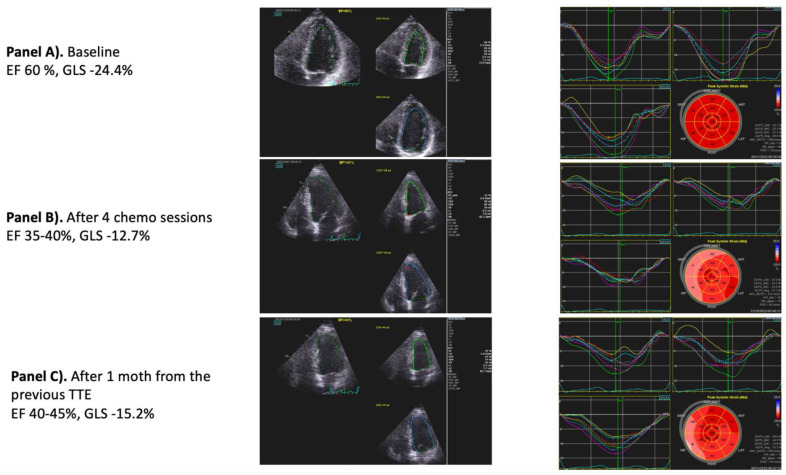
Echocardiography in the evaluation of chemotherapy CV side-effects. A 58-year-old female patient with known dyslipidemia and no other cardiovascular disease (Panel A). The patient was affected by stage IIIC high-grade serous ovarian cancer. She underwent four sessions of taxol/carboplatin protocol (S/P 4 cycles) before surgical debulking. After the last chemo session, she developed shortness of breath during mild physical effort which she never experienced before. At TTE, a significant reduction in LVEF and GLS compared with the baseline was seen (Panel B). The patient was put on metoprolol and Lisinopril. Atorvastatin and TTE were repeated after 1 month with evidence of mild improvement in LVEF and GLS (Panel C). Abbreviations: EF, left ventricular ejection function; GLS, global longitudinal strain; TEE, transthoracic echocardiography.
